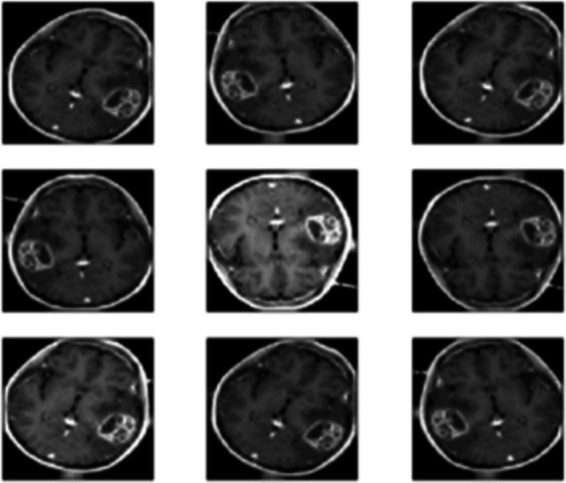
Augmentation of MRI images.
